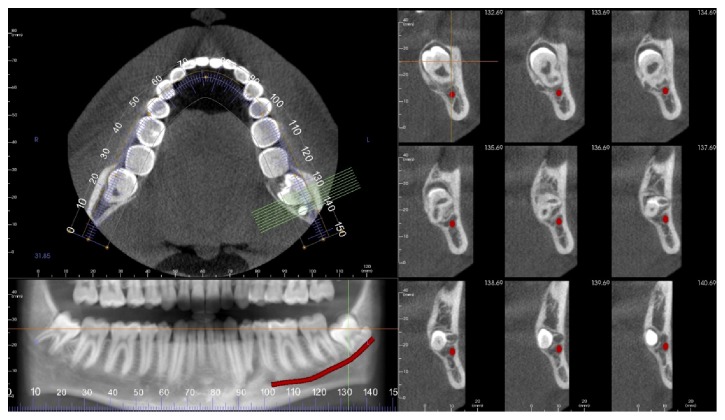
Panoramic radiograph and cross-sectional view proximity of the inferior canal to concrescence tooth.
